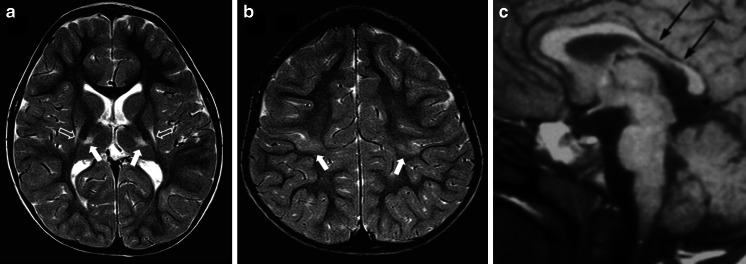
MR images in a 6-year old boy with dyskinetic cerebral palsy who has the typical neuroimaging findings of acute profound hypoxic–ischaemic brain injury. a, b Axial T2-weighted MR images at the level of the basal ganglia (a) and peri-rolandic cortex (b) show gliosis and reduction in volume of the putamina (*open arrows*), thalami and paracentral lobules (*solid arrows*). c A mid-sagittal T1-weighted MR image shows a focal reduction in thickness in the posterior part of the body of the corpus callosum (*arrows*)
